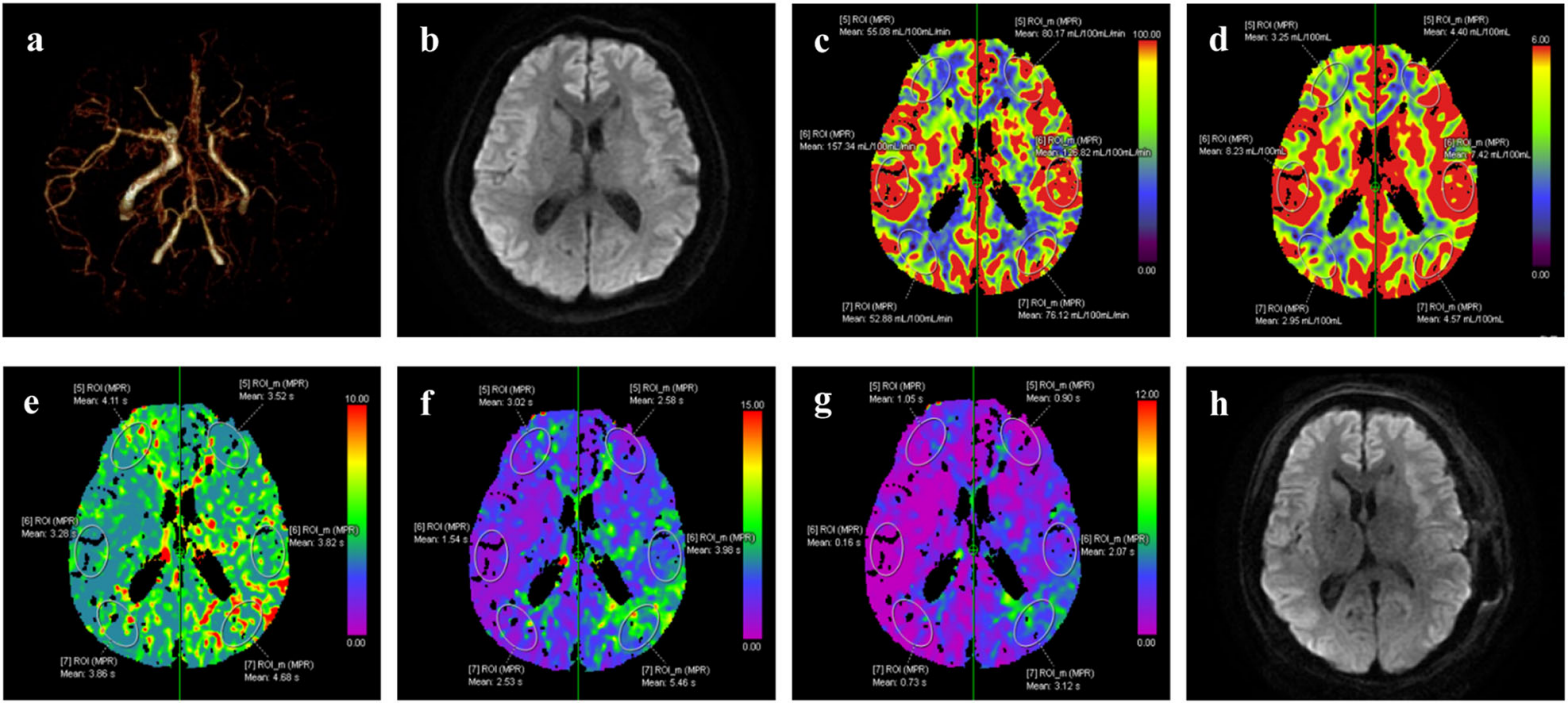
A 50-year-old male MMD patient without post-CI. Preoperative CTA showed MMD (a). Preoperative diffusion-weighted imaging showed no acute cerebral infarction (b). Preoperative CTP showed no obvious changes in CBF (c), CBV (d), and a mild increase in MTT (e), TTD (f), and Tmax (g) in the left temporal-parietal lobe and right frontal lobe. Postoperative diffusion-weighted imaging showed no cerebral infarction (h)
